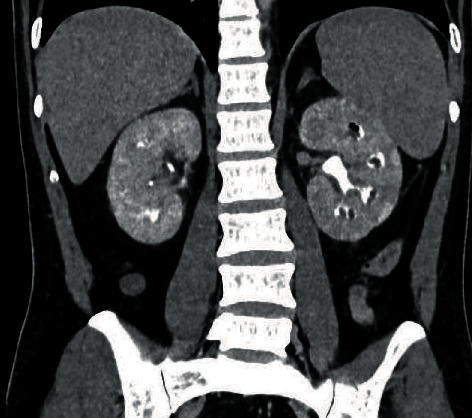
Renal papillary necrosis in the left kidney on delayed contrast CT scan on the 5^th^ postoperative day. Coronal reconstruction. Air in the upper caliceal system represents prior instrumentation.
